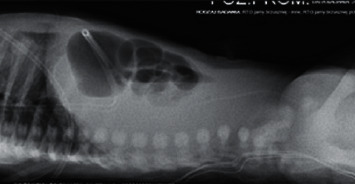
Abdominal X-ray on the first day of life—dilated stomach and bowel loops.
